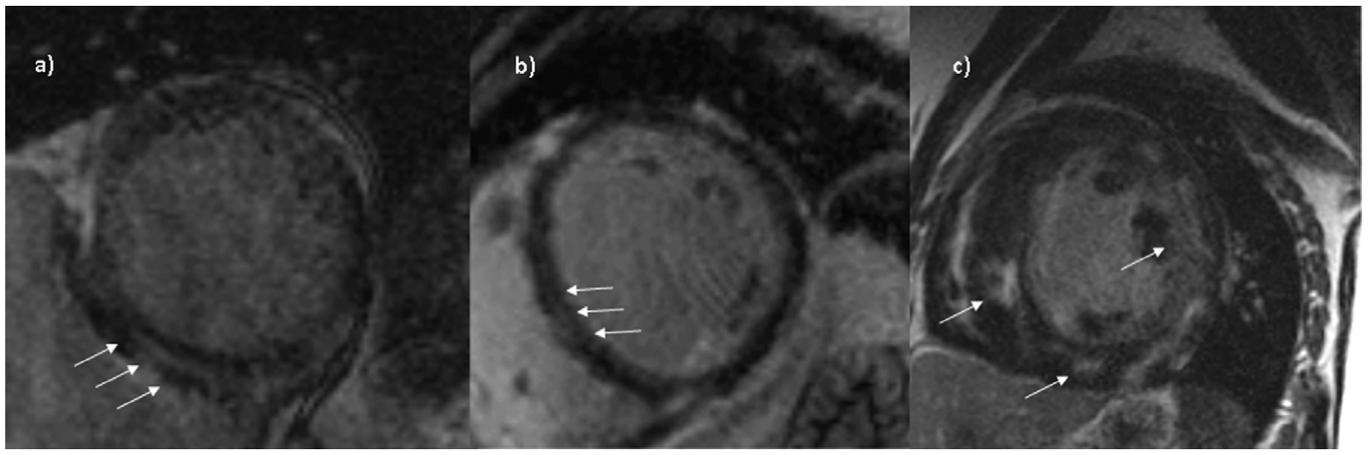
Late gadolinium-enhanced (LGE) cardiac magnetic resonance imaging in short-axis orientation of the left ventricle in three different patients. Different types of LGE can be found, characteristic for entity of non-ischemic cardiomyopathy. (a) Epicardial LGE in a patient suspected of having (sub)acute myocarditis. (b) Non-ischemic cardiomyopathy with diffuse midwall stripe pattern of the septum, indicating idiopathic dilated cardiomyopathy. (c) Typical patchy LGE/fibrosis of the septal as well as the free lateral wall segments seen in hypertrophic cardiomyopathy.
